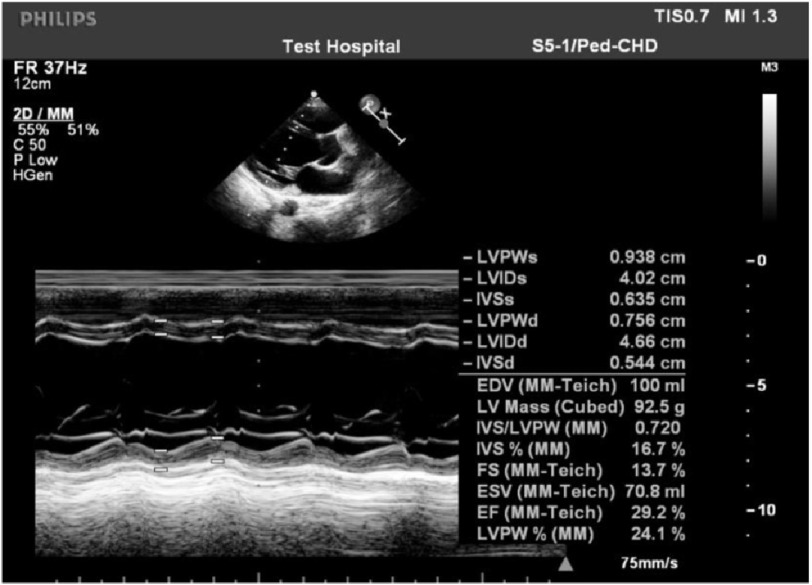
TTE, parasternal long-axis view: dilated LV dimensions and impaired LV systolic function.
